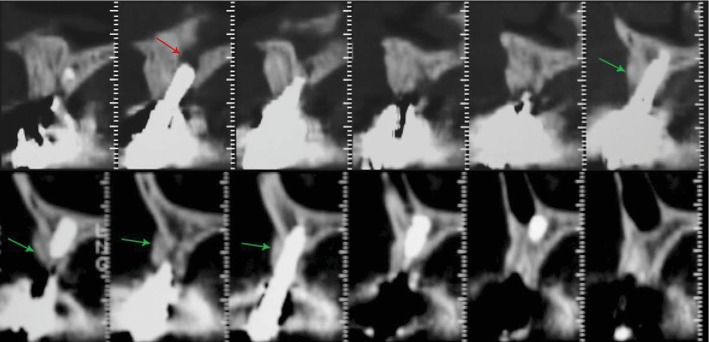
Computed tomography scans showed the implant in the region of the central incisor inside the incisive canal (red arrow) and the three-dimensional bone loss around the implants, which were osseointegrated only in the apical portion with the remaining surfaces being covered by radiopaque biomaterial particles encapsulated in the soft tissues (green arrows).
